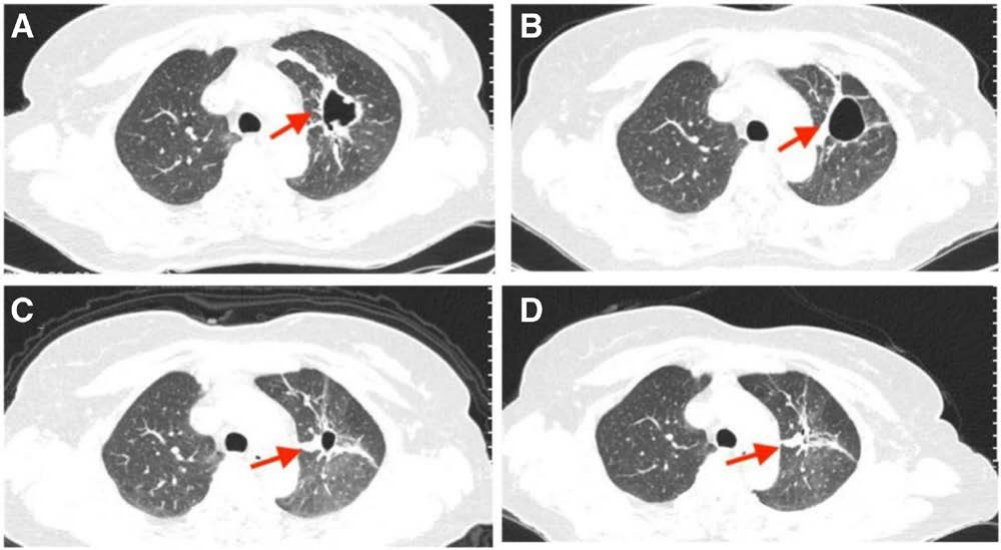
Patient 1: Chest CT images at a different stage. The major lesion (red arrow) is in the superior lobe of the right lung. (A) Before immunotherapy on June 17, 2020. (B) After 4 cycles of combination immunotherapy on October 5, 2020. (C) A pancreatic lesion was found on March 9, 2021, and chest CT scanning showed persistent remission of the pulmonary lesion. (D) Chest CT image on June 9, 2021 showed persistent remission of the pulmonary lesion. CT = computed tomography.
